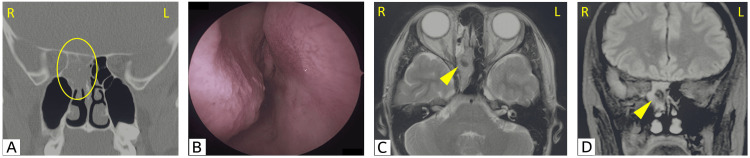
Findings on admission (A) Paranasal sinus CT image obtained at the initial visit. A soft tissue shadow with partial calcification was observed in the right sphenoid sinus and the right posterior ethmoid sinus (circle). No bone destruction was observed. (B) Nasal endoscopy findings at the initial visit. No purulent discharge or mucosal edema was observed. (C, D) Paranasal sinus MRI (C, T2-weighted image; D, FLAIR image) at the initial visit revealed a lesion in the right sphenoid sinus and the right posterior ethmoid sinus with low signal intensity (arrowheads). FLAIR: Fluid-attenuated inversion recovery
